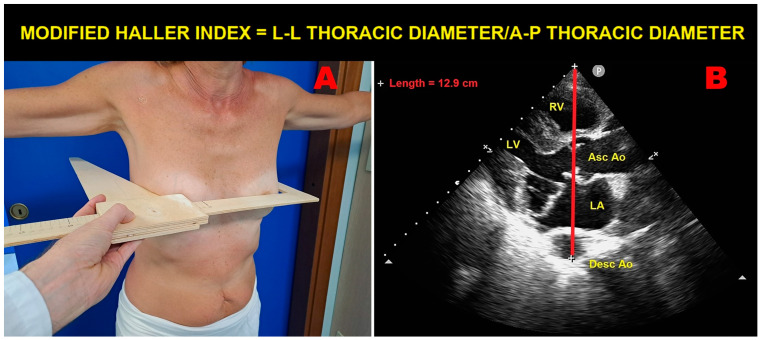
An example of modified Haller index assessment in an individual with mitral valve prolapse. Panel (A): The L-L thoracic diameter, measured with the individual in the standing position and with open arms, using a rigid ruler in centimeters coupled to a level (the measuring device) placed at the distal third of the sternum at the point of maximum depression of the sternum. Panel (B): The A-P thoracic diameter, obtained with the individual in the left-lateral decubitus position during conventional transthoracic echocardiography by placing a 2.5 mHz transducer near the sternum in the left third or fourth intercostal space to obtain a parasternal long-axis view, and measuring the distance between the true apex of the sector and the anterior surface of the vertebral body. The vertebral body is identified by using, as a reference point, the posterior wall of the descending thoracic aorta, visualized behind the left atrium. Ao, aorta; A-P, antero-posterior; Asc, ascending; Desc, descending; LA; left atrium; L-L, latero-lateral; LV, left ventricle; RV, right ventricle.
